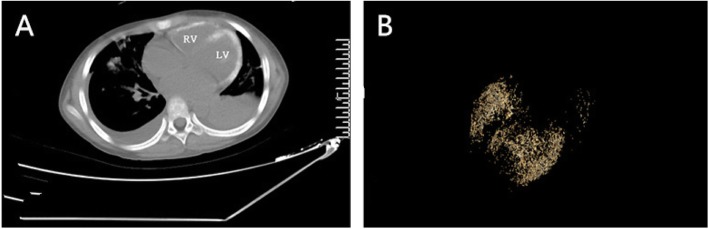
a Routine computed tomography(CT) scan of the heart showing extensive calcification of both ventricular walls and chordae on the 14th day; b Revolution CT scanning of the myocardial calcification
